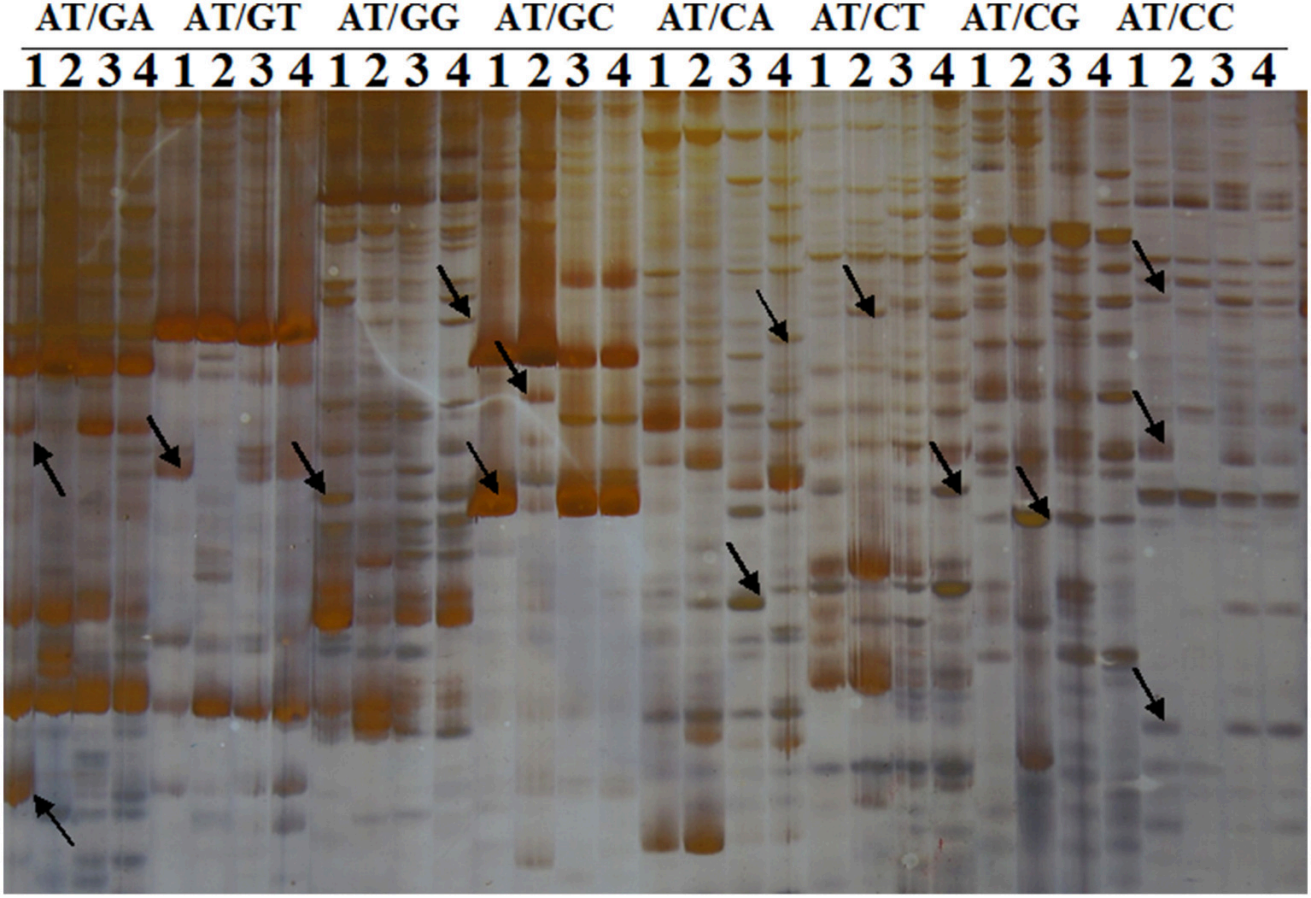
cDNA-AFLP profiles using one *Eco*R I selective primer and eight *Mes* I selective primers. One *Eco*R I selective primer: *Eco*R I-AT; Eight *Mes* I selective primers: *Mes* I-GA, GA, GG, GC, CA, CT, CG, and CC. 1, Control roots of *C. grandis*; 2, Excess B-treated roots of *C. grandis*; 3, Control roots of *C. sinensis*; 4, Excess B-treated roots of *C. sinensis*. Arrows indicate differentially expressed TDFs.
